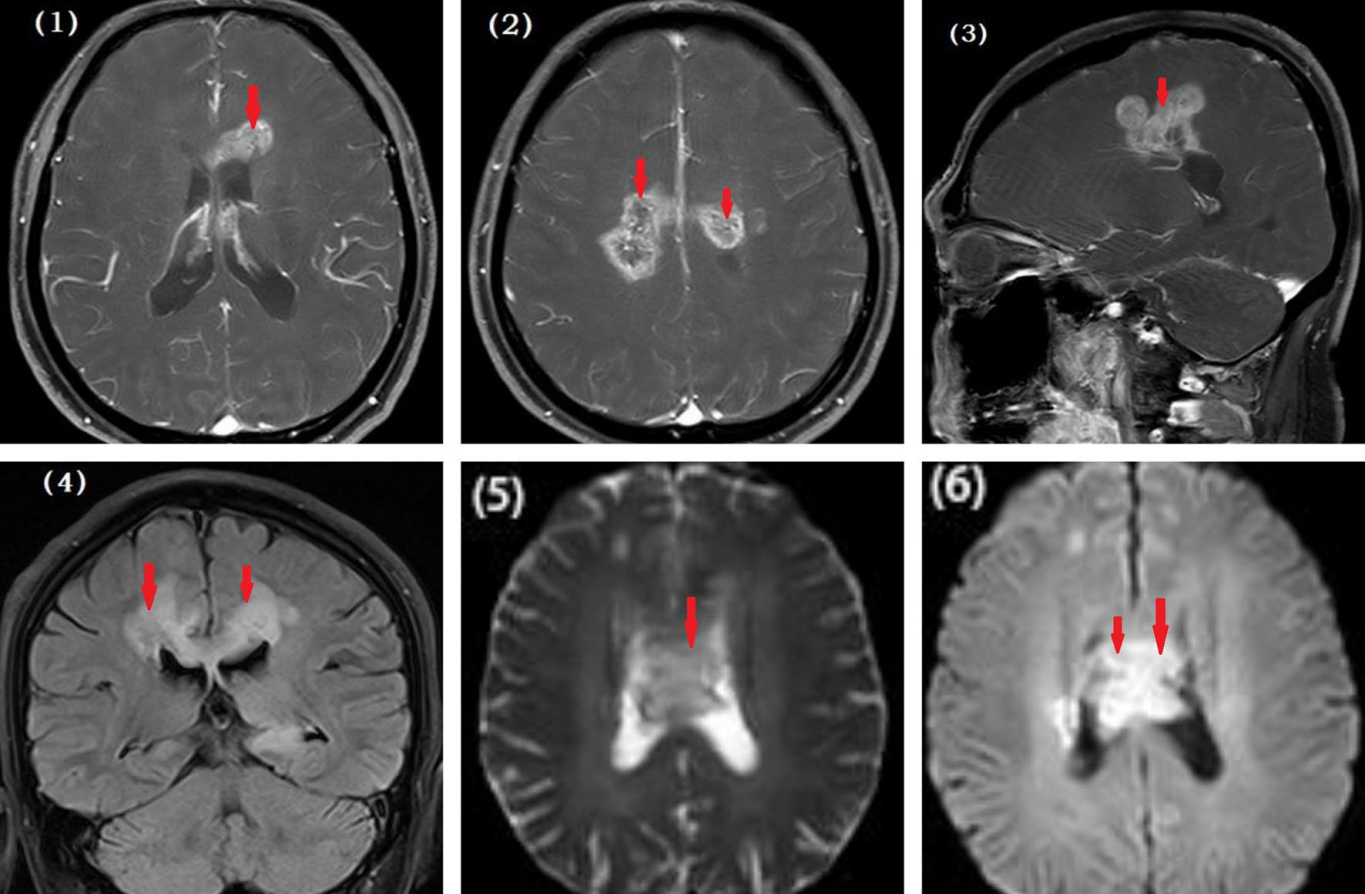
Magnetic resonance imaging (MRI) of the head. (1–4) Abnormal lesions are visible in the corpus callosum, semi-oval centers, and the area below the frontal cortex. Contrast-enhanced MRI of the head shows enhanced intracranial lesions within the same areas. The lesions were hyperintense on fluid-attenuated inversion recovery and hypointense on diffusion-weighted imaging (5, 6)
